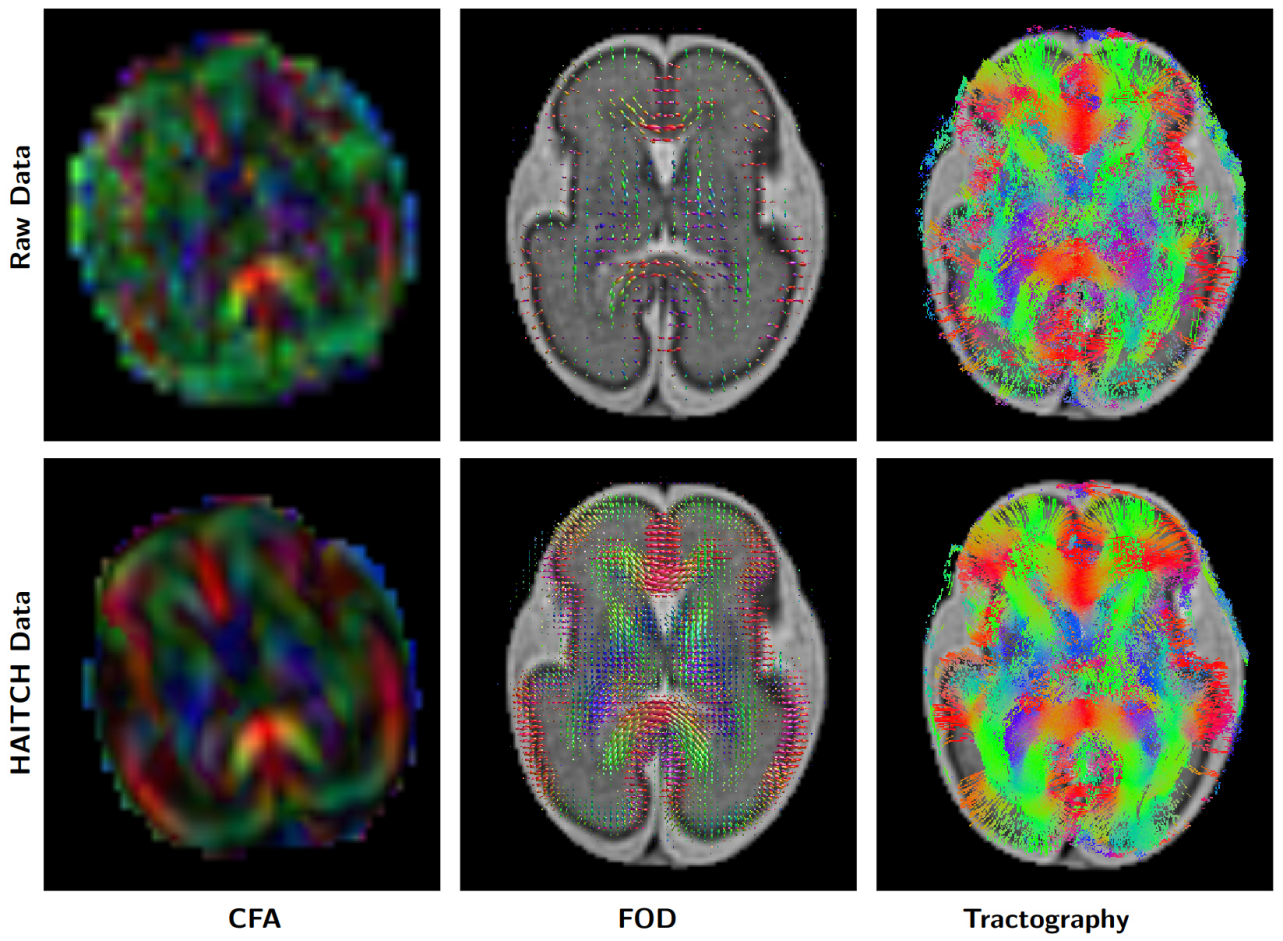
Comparison of Anatomical Accuracy Before and After HAITCH Correction. The top row shows the raw data, while the bottom row shows the HAITCH-corrected data. From left to right: Colored Fractional Anisotropy (CFA) maps, Fiber Orientation Distributions (FOD), and tractography streamlines. The FODs are overlaid on a T2-weighted image in the middle column, and the tractography streamlines are shown in the right column. The images depict the brain of a 30-week fetus, with improved alignment of the white matter pathways after motion correction, demonstrating the efficacy of HAITCH in reducing artifacts and enhancing anatomical accuracy.
